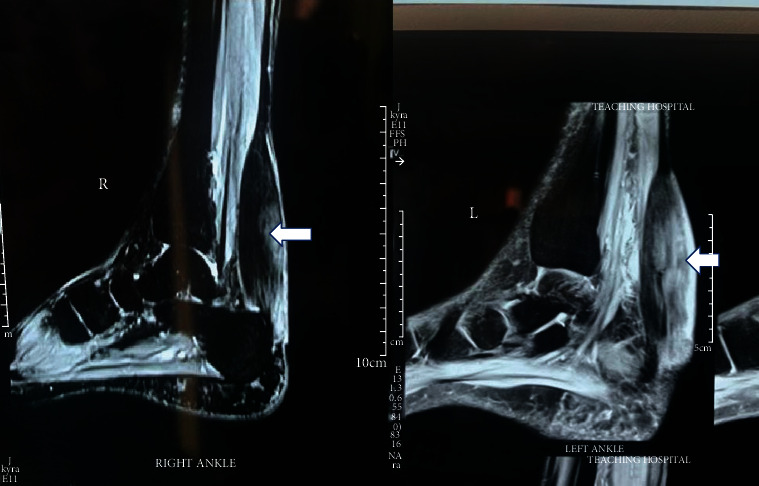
MRI scan of the lower limbs. Sagittal view: right (R) and left (L) tendon xanthomas (white arrows).
